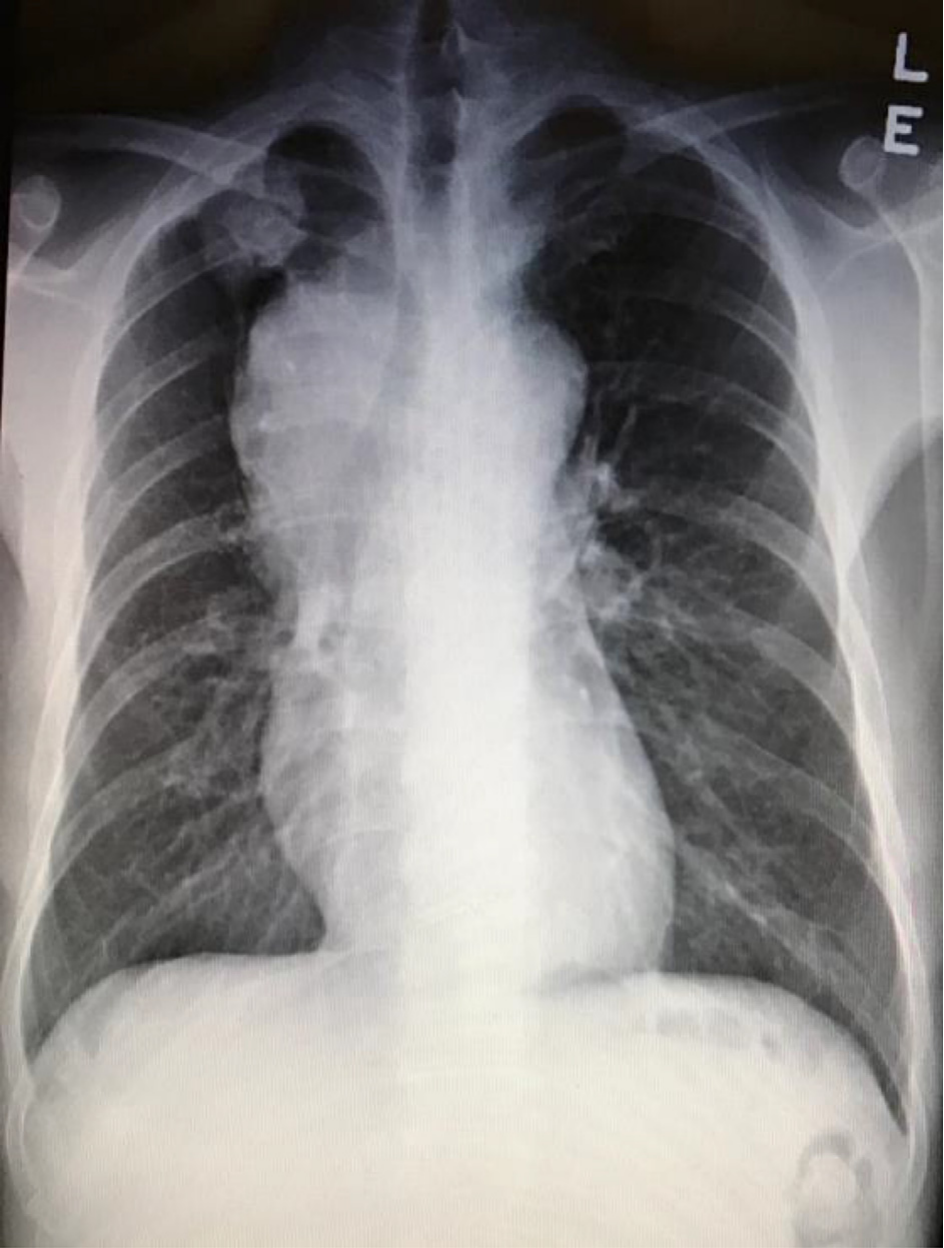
Chest radiograph at presentation.
